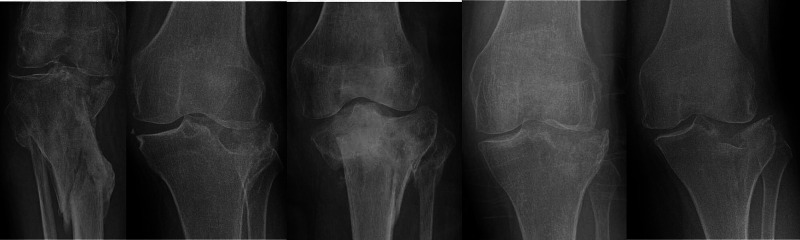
Anterior-posterior radiographs four months following the injury. Case one to five (left to right).
